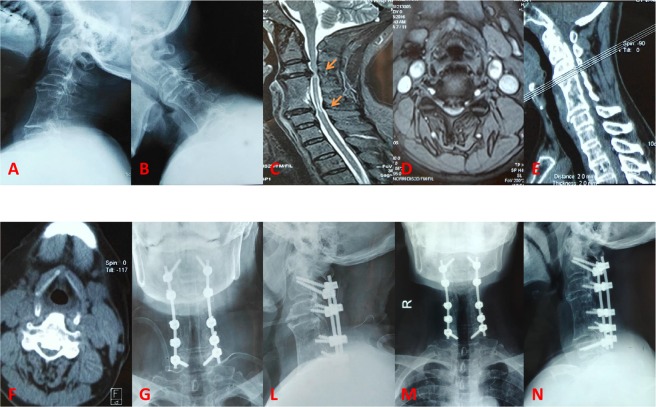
Radiological images preoperatively and postoperatively. (A,B) preoperative X-ray images; (C,D) preoperative MRI scan; (E,F) preoperative CT scan; (G,L) postoperative X-ray immediately; (M,N) postoperative X-ray at final follow-up.
